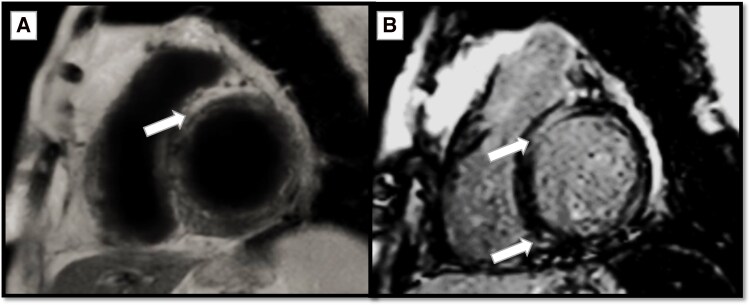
(*A*) Short-axis view T2-weighted image showing increased signal intensity of the basal anteroseptal segment; indicative of myocardial oedema (thick arrow). (*B*) Short-axis view late gadolinium enhancement image showing hyperenhancement of multiple myocardial segments; indicative of myocardial fibrosis (thick arrows). Both findings fulfil the original Lake Louise criteria of active myocarditis.
